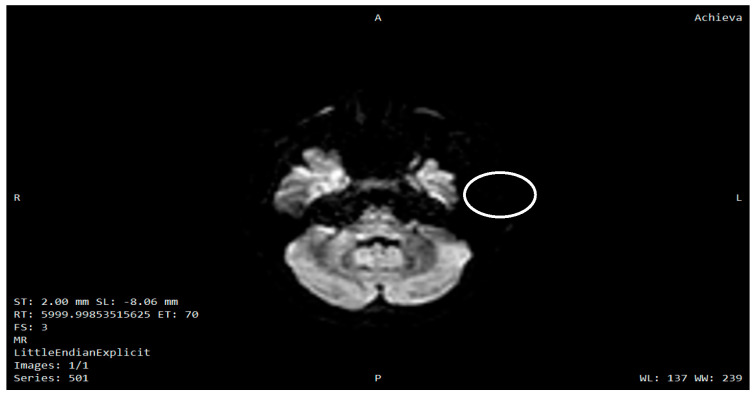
Axial diffusion-weighted MRI showing marked involvement of the pons (circled) in a patient with genetically confirmed Wolfram syndrome. This brainstem lesion pattern is characteristic of the neurodegenerative process described in the disease. A = anterior; P = posterior; R = right; L = left.
